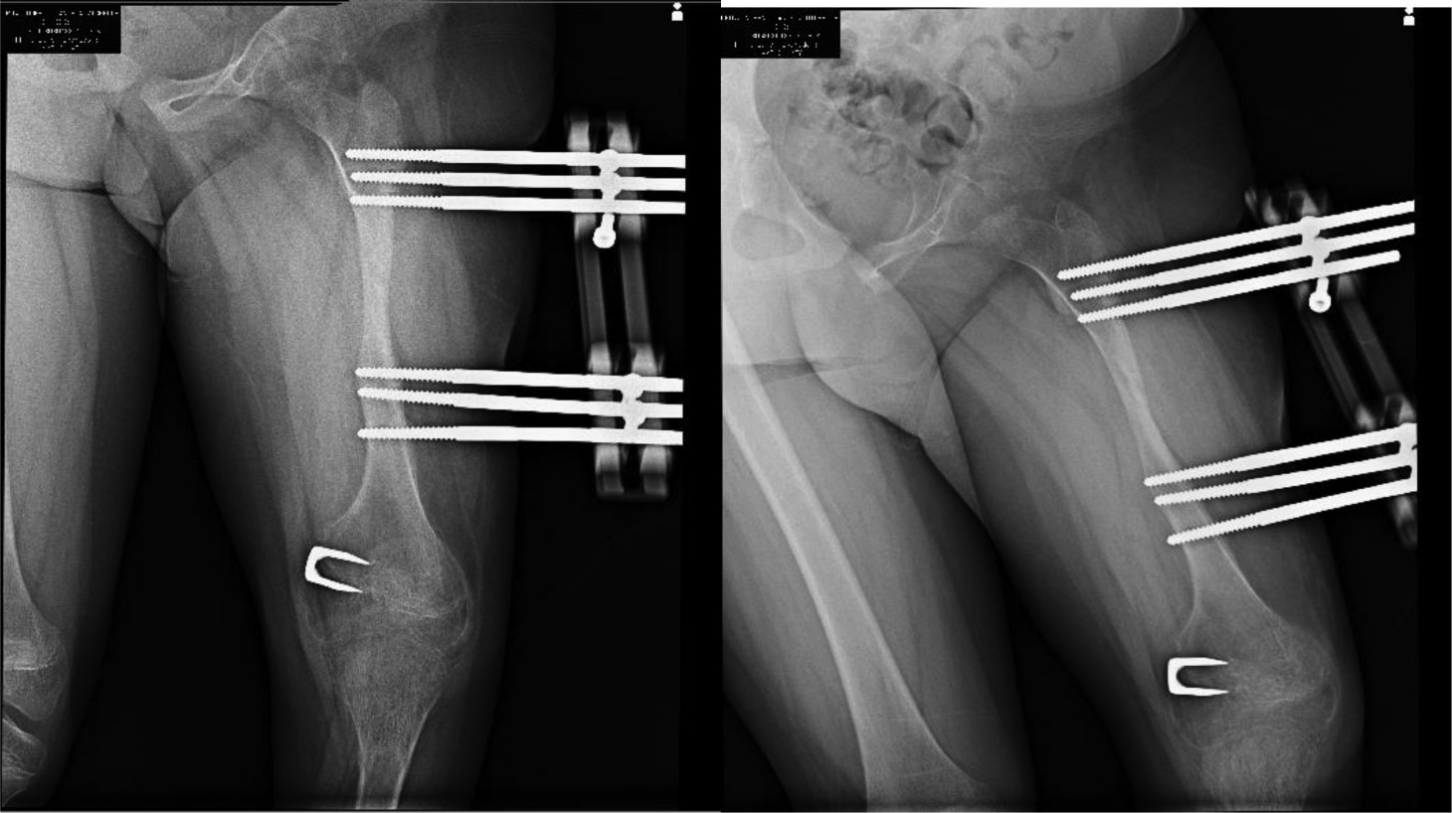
Six months postoperative anteroposterior and lateral radiographs of the left femur. Femur lengthening employing uniplanar straight-line external fixation and lengthening of 6.7 cm femoral length.
